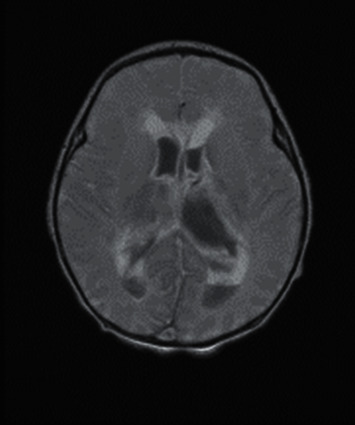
Magnetic resonance imaging of the brain showing intraventricular hemorrhage within the lateral third and fourth ventricles with hydrocephalus. Bilateral thalami and basal ganglia hemorrhage can be noted, primarily on the left side. Diffuse diffusion signal abnormality can be noted in the cerebral hemisphere, likely representing ischemic/necrotic changes.
